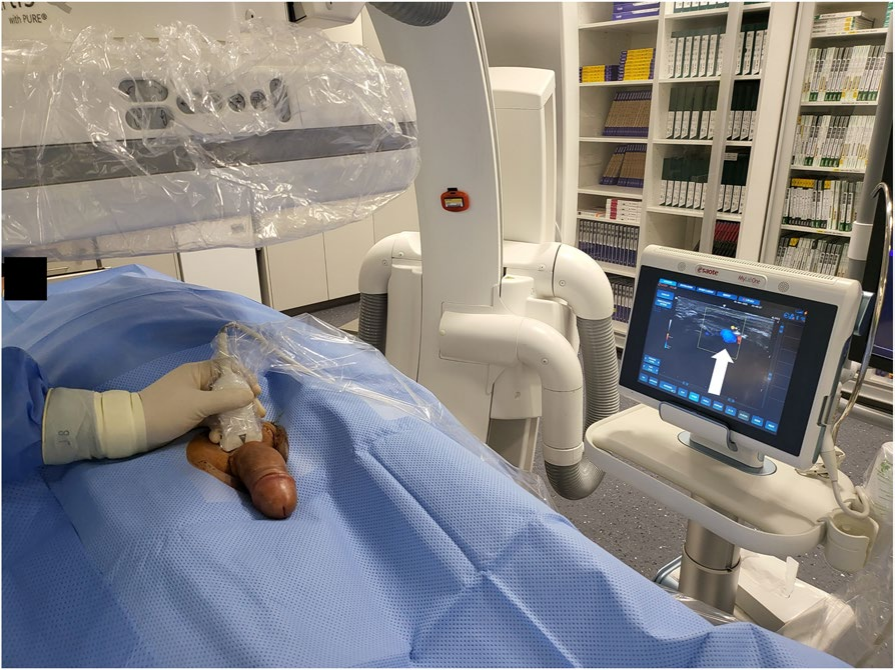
Angiosuite setup with fluoroscopy and ultrasound. This patient’s penis is sterilely prepped and draped. A 9 MHz ultrasound probe is also sterilely draped and color duplex is used to localize a proximal deep dorsal penile vein (arrow)
